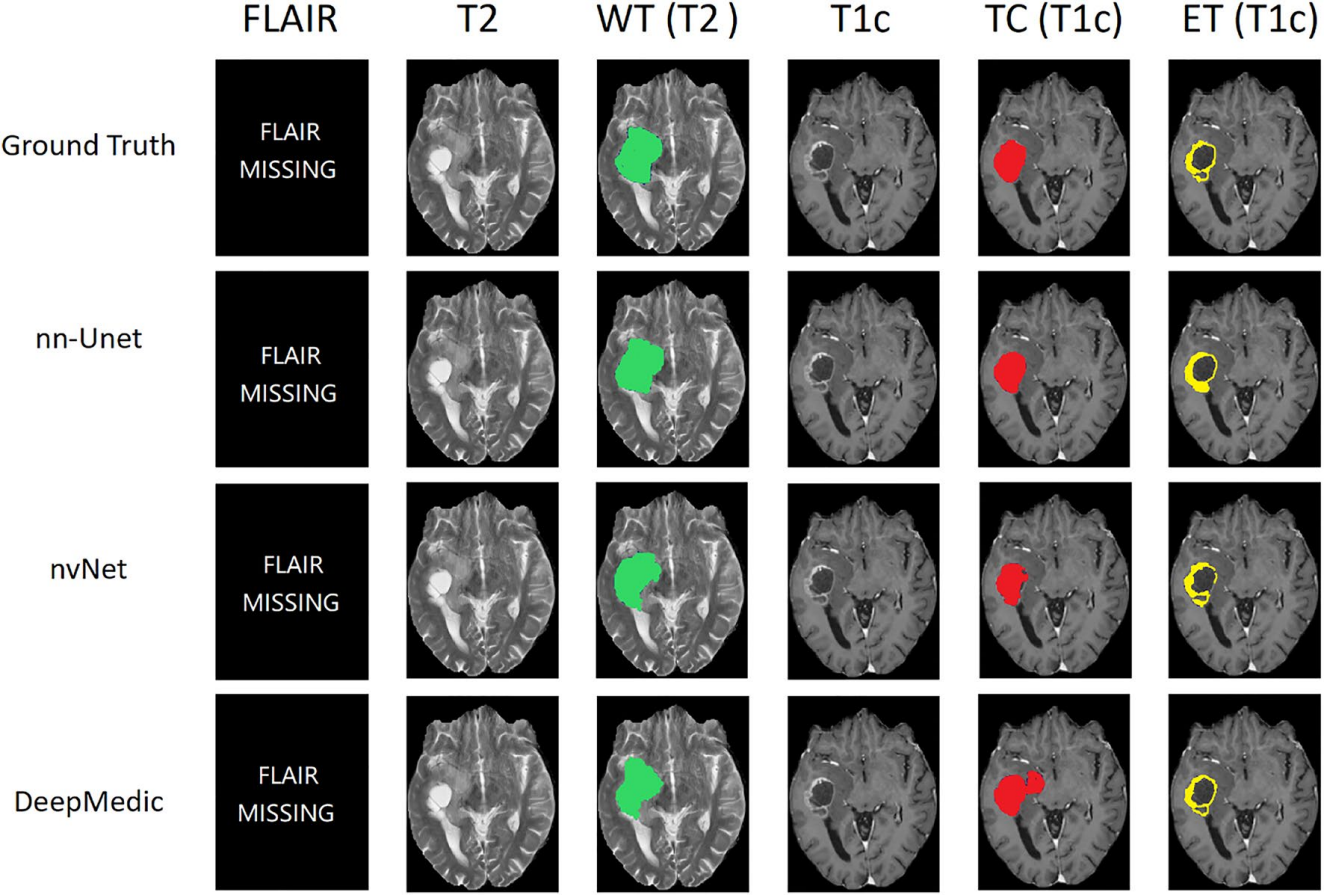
GBM patient from the PICTURE dataset with missing FLAIR scan. Whole Tumor (WT—green) is the full extent of the tumor, including the tumor core, non-enhancing tumor and oedema, indicated by hyperintensity on FLAIR and T2w. Tumor Core (TC—red) is the main body of the tumor and most likely area of resection. The TC includes the enhancing tumor (ET—yellow) and necrosis. DSCs in this case for nn-Unet were WT = 0.93, TC = 0.94, ET = 0.83; nvNet WT = 0.89, TC = 0.92, ET = 0.80; and DeepMedic WT = 0.81, TC = 0.85, ET = 0.81.
